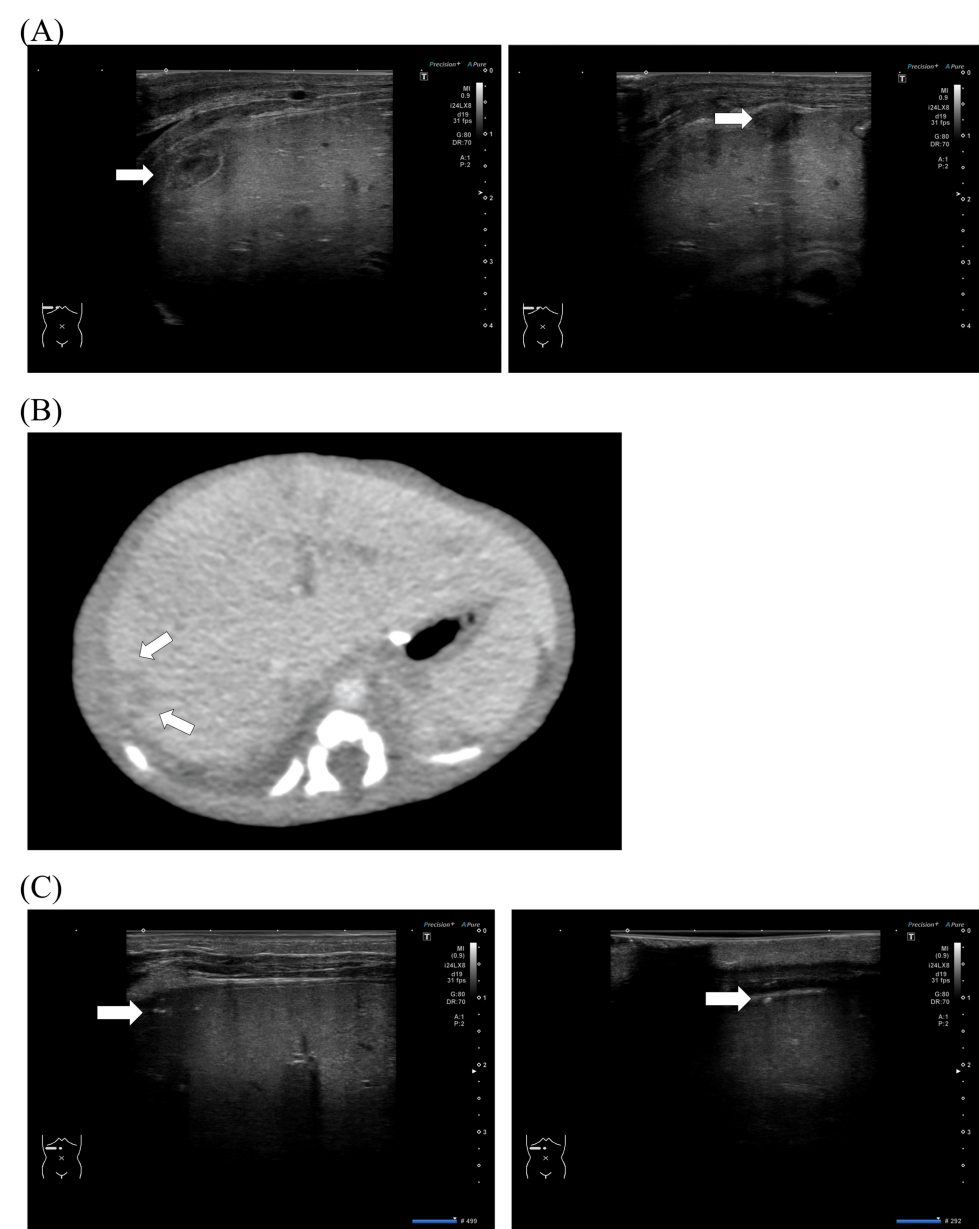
Ultrasound and computed tomography images of liver abscesses during the course of the disease. (A) Abdominal ultrasound performed on day 23 of life. Liver abscesses were detected in the liver parenchyma (arrow), with one in segment S7 (left) and the other in S8 (right) of the hepatic zone, measuring 20 mm and 5 mm in diameter, respectively. The irregular, low-echogenic heterogeneous lesions with irregular margins were consistent with abscesses. (B) Abdominal contrast-enhanced computed tomography (CT) performed on day 26 of life. CT indicated only a single low-density 15 mm nodule (arrow), while four lesions were observed on the abdominal ultrasound performed on the same day. (C) Abdominal ultrasound performed on day 79 of life. Liver abscesses in segments S7 (left) and S8 (right) had shrunk and became organized (arrow).
